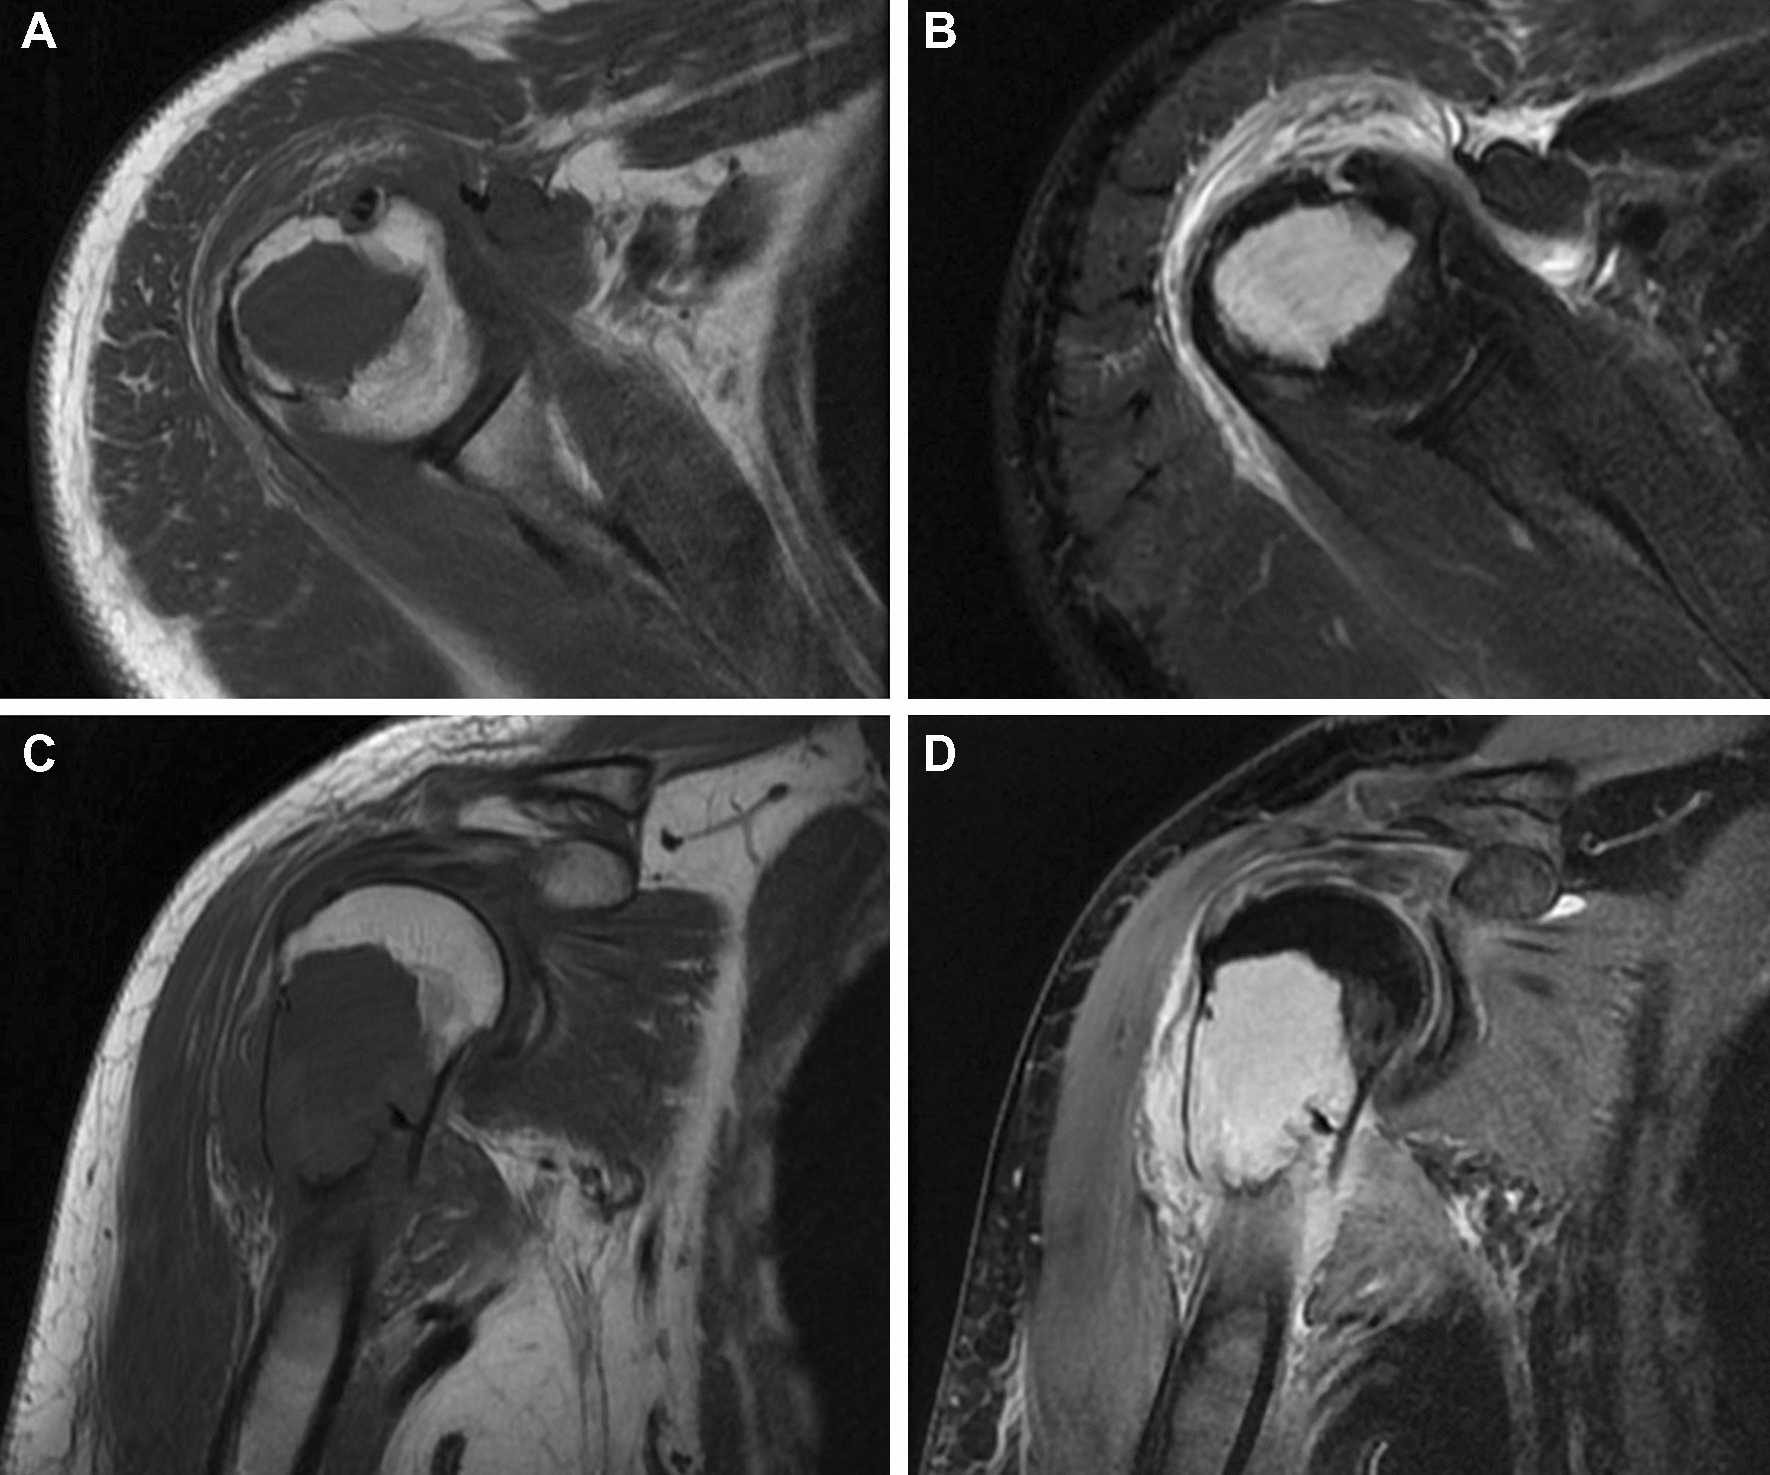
T1-weighted image shows an intramedullary lesion, isointense to muscle, A axial image, C coronal image. T2-weighted image shows a hyperintense lesion associated with soft tissue edema, B axial image, D coronal image
